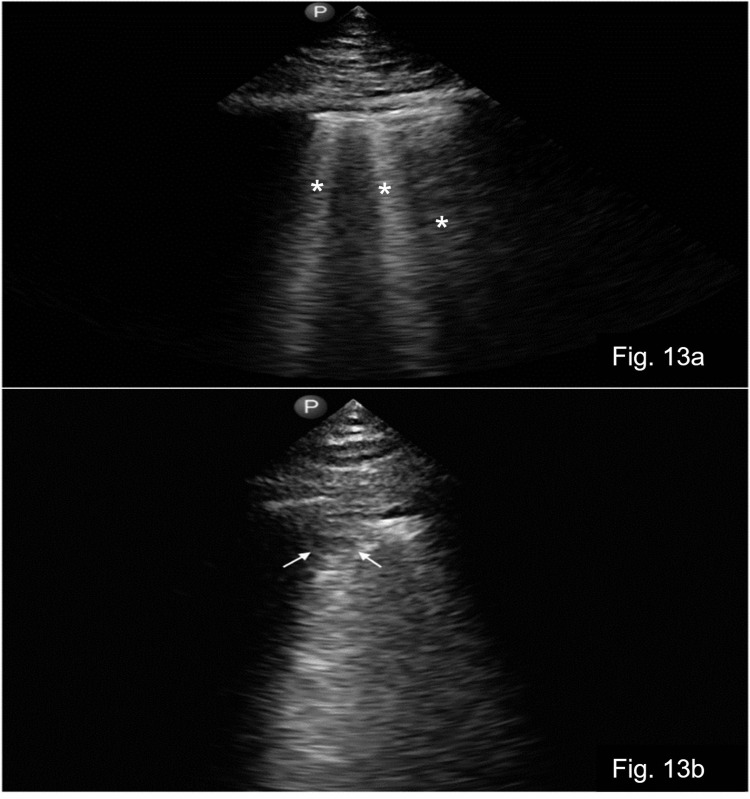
Lung ultrasound images 13a: Confluent B lines on lung ultrasound indicating fluid within the lung. 13b: White arrows indicating subpleural consolidations on lung ultrasound.
